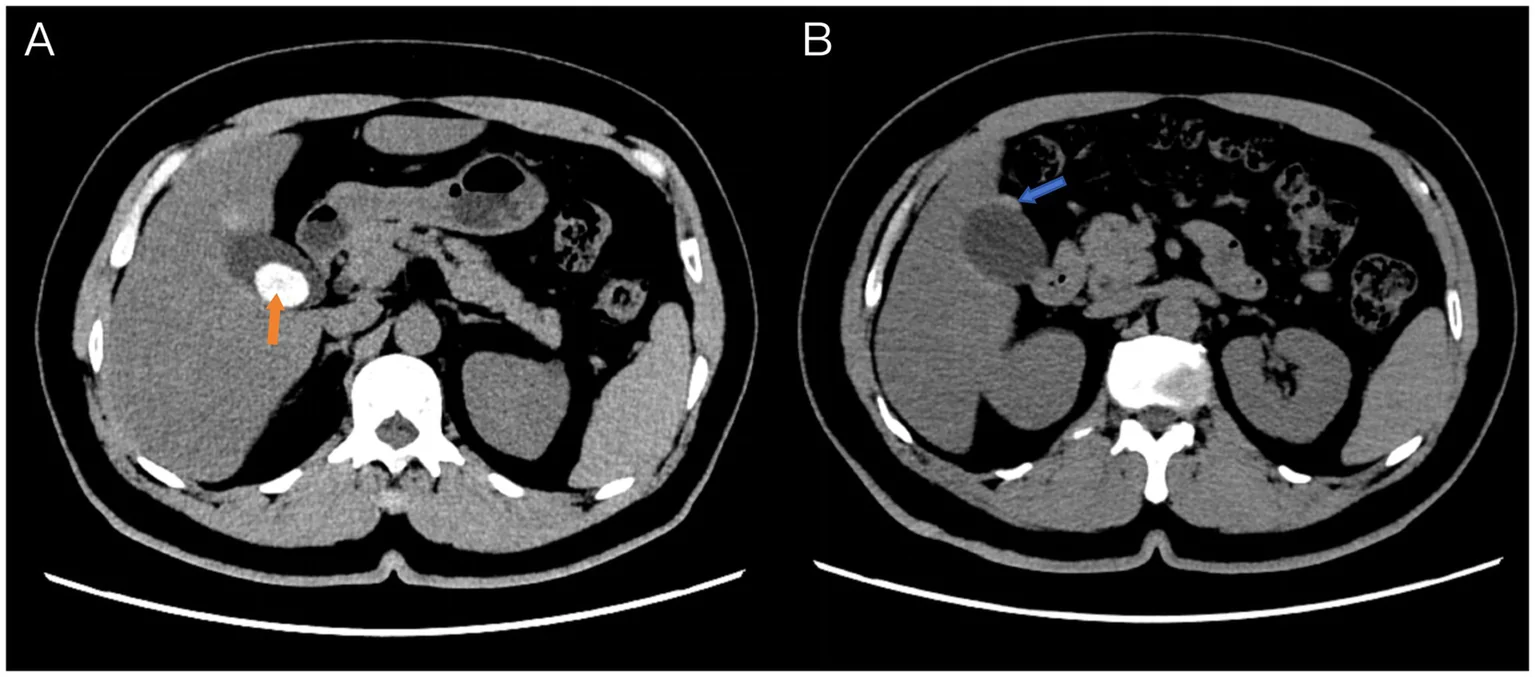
Preoperative computed tomography (CT) findings of the gallbladder and an exophytic nodular (A) A hyperdense lesion (orange arrow) is noted within the gallbladder lumen, consistent with a gallstone. (B) Unenhanced CT image shows an exophytic nodular (blue arrow) attached to the serosal surface of the anterior wall near the fundus, exhibiting a homogeneous density isodense to the native liver.
